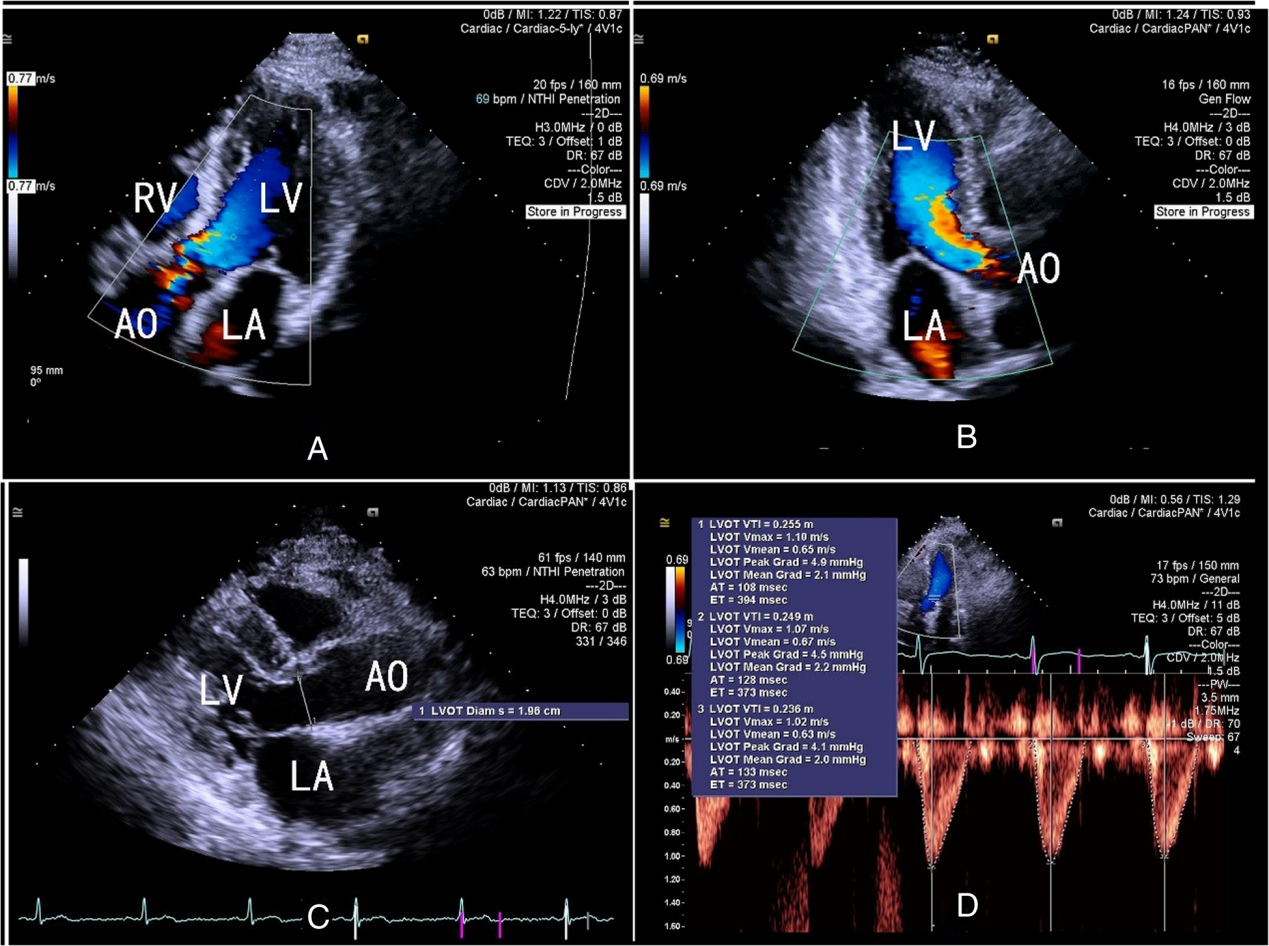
CO assessment using 2D-PWD transthoracic echocardiography. Cardiac output was calculated using the LVOT diameter, LVOT VTI, and heart rate. In routine practice, LVOT velocity–time integrals (VTIs) could be acquired in the apical five chamber view (panel a) or apical long axis view (panel b). Diameters of the left ventricular outflow tract (LVOT) were measured in the parasternal left ventricular long axis view during the early phase of ventricular systole (panel c). LVOT VTI was automatically measured for three consecutive beats and averaged (panel d). RV, right ventricle; LV, left ventricle; LA, left atrium; AO, aorta
